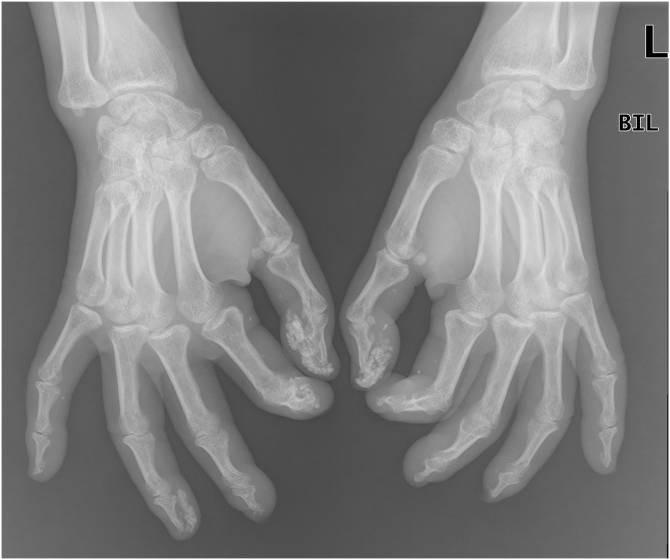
Hands simple radiography (oblique view) before treatment with intralesional sodium thiosulfate.
